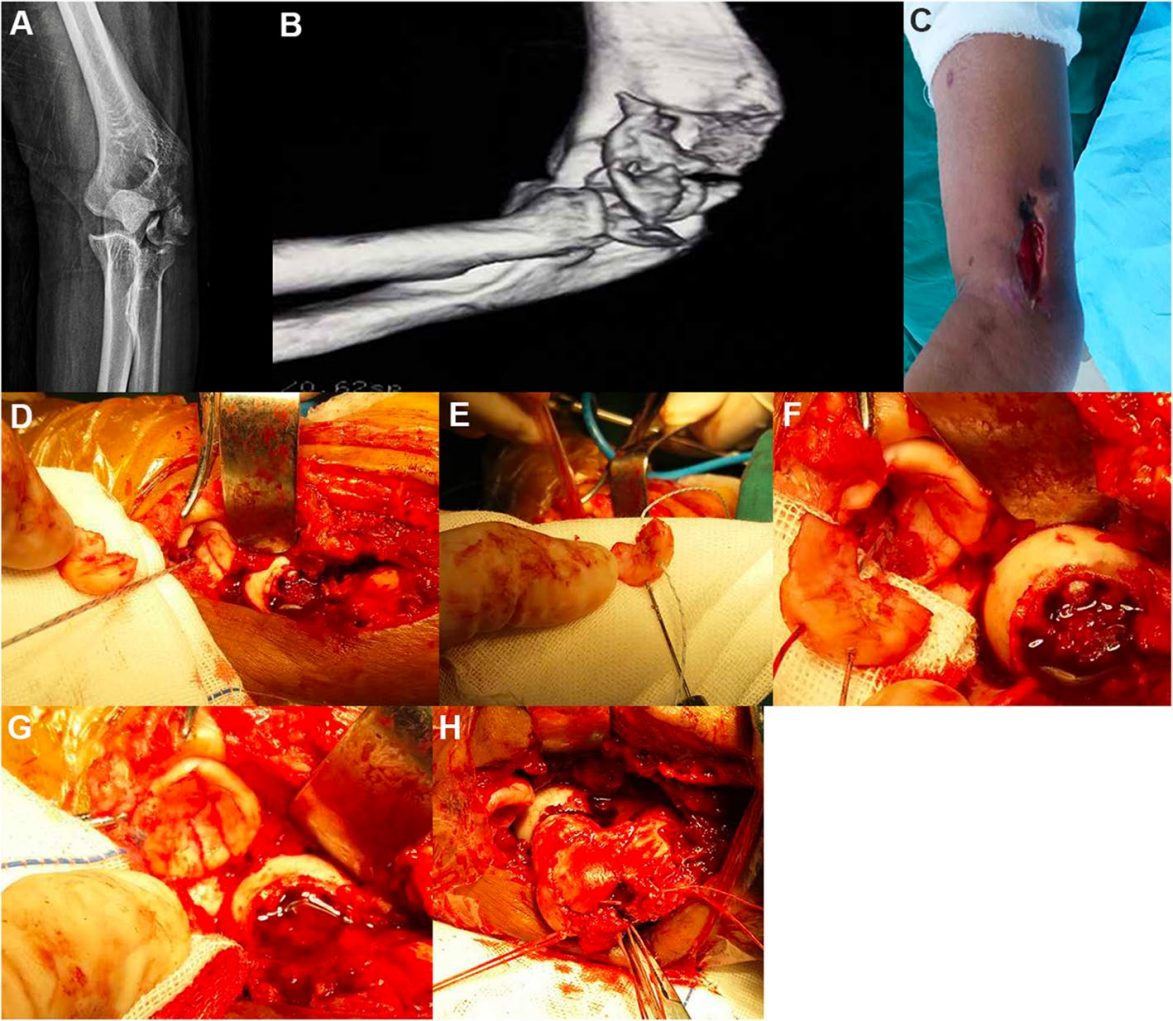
Case 2: A preoperative X-ray (A) and spiral CT scan (B) reveal a severe comminuted fracture of the radial head (type IV), severe comminution, and dislocation of the humeral capitulum and the trochlea (Bryan and Morrey type IV). During the operation, the incision (C) is extended downward from the original wound, with a total length of 12 cm, and the exploration reveals that the articular surface of the radial head is comminuted into four pieces and the subchondral bone has collapsed. A 2.8-mm anchor is fixed in the stronger area of the medullary cavity, the tail thread is led out from the broken end of the fracture (D), and the two tail threads of the anchor respectively run in parallel through the freed bone block (E and F). After the reduction is satisfactory, the two tail threads are tightened and made into a knot at the radial neck (G). For the comminuted fractures of the capitulum humeri and the lateral trochlea, similarly, two 3.5-mm anchors are screwed into the bone bed section of the medial semi trochlea, then 8 tail threads of the anchors are run through all freed bone blocks horizontally (H). After satisfactory reduction, the threads were tightened and made into knots around the lateral epicondyle of the humerus
